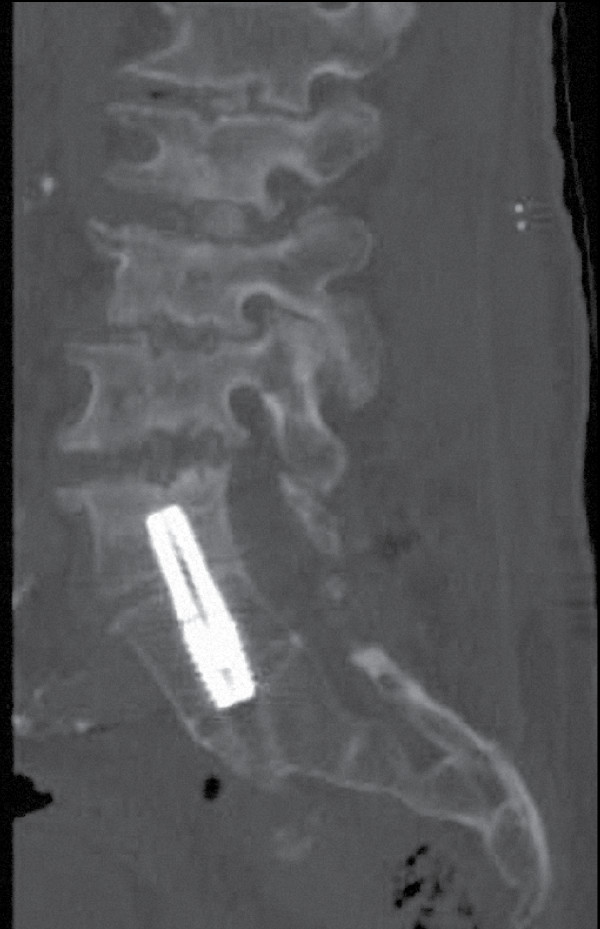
Sagittal computed tomography (CT) image following successful revision and replacement of the AxiaLIF rod. Note re-establishment of L5-S1 disc space distraction with improvement of radicular symptoms.
